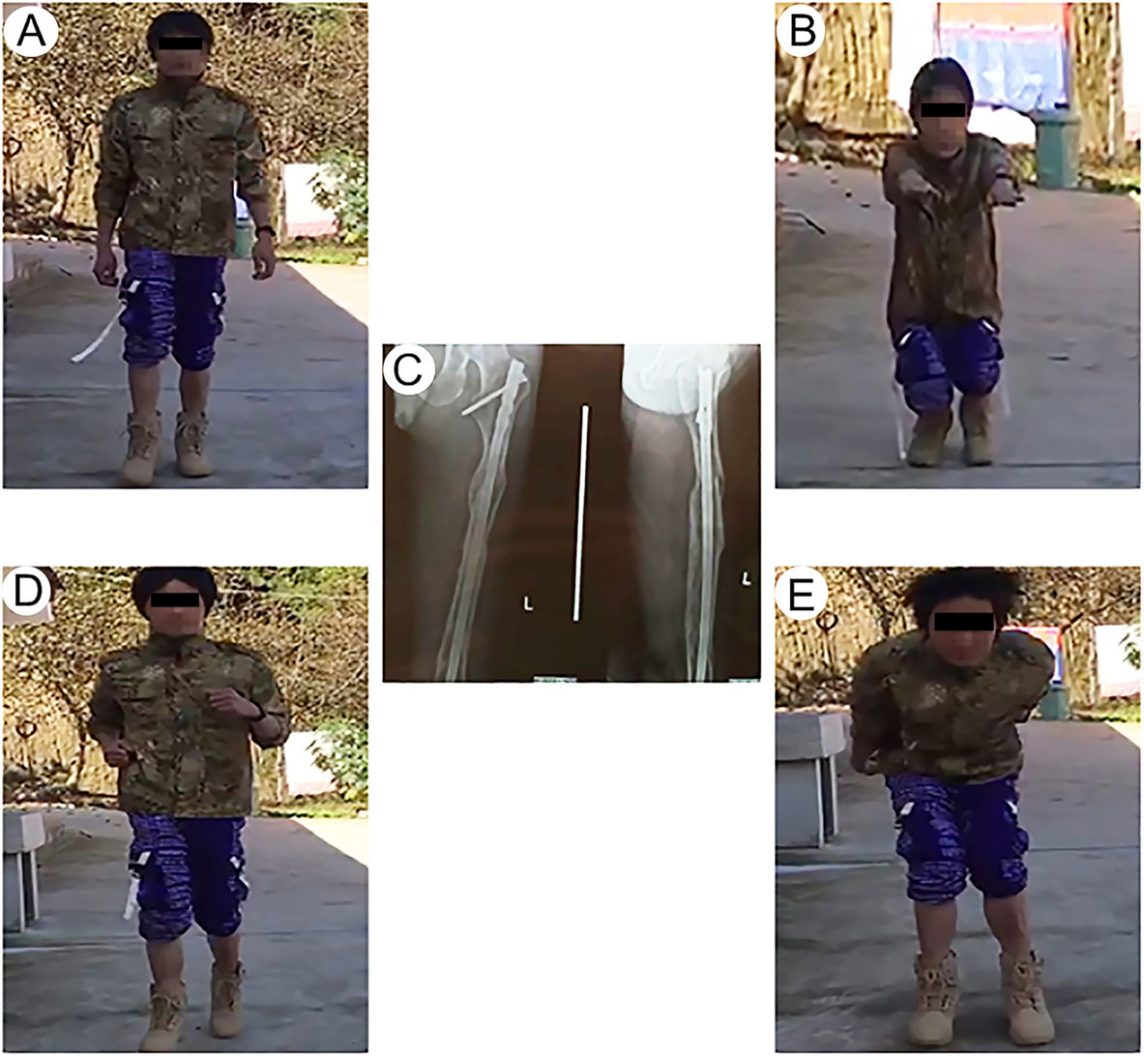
X-ray radiograph showing the fracture after 12 months with complete callus bridging the fracture (c) and full functional range of motion when walking (a), squatting (b), running (d), jumping (e) with full weight-bearing
